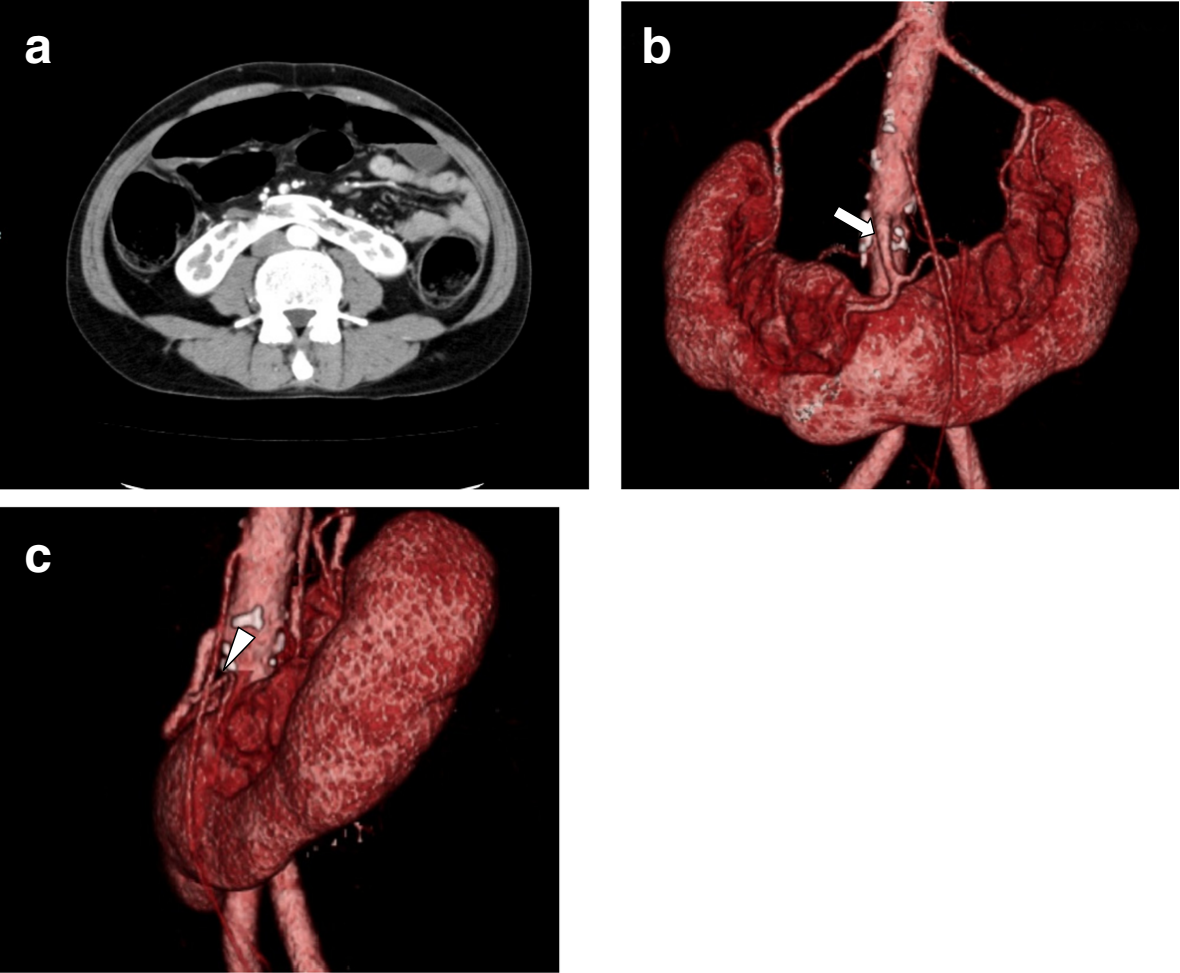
a Abdominal CT showing horseshoe kidney. b, c 3D-CT angiography shows the root of an aberrant renal artery (white arrow) at the isthmus from 3 cm under the IMA branch of the aorta, and the left branch of aberrant renal artery (arrowhead) was close to IMA
